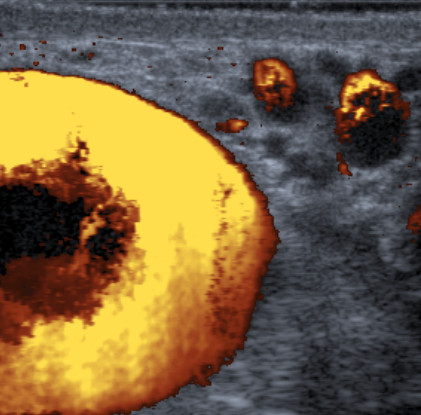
Colour Doppler ultrasound image – flow demonstrated in fluid surrounding left testis.
